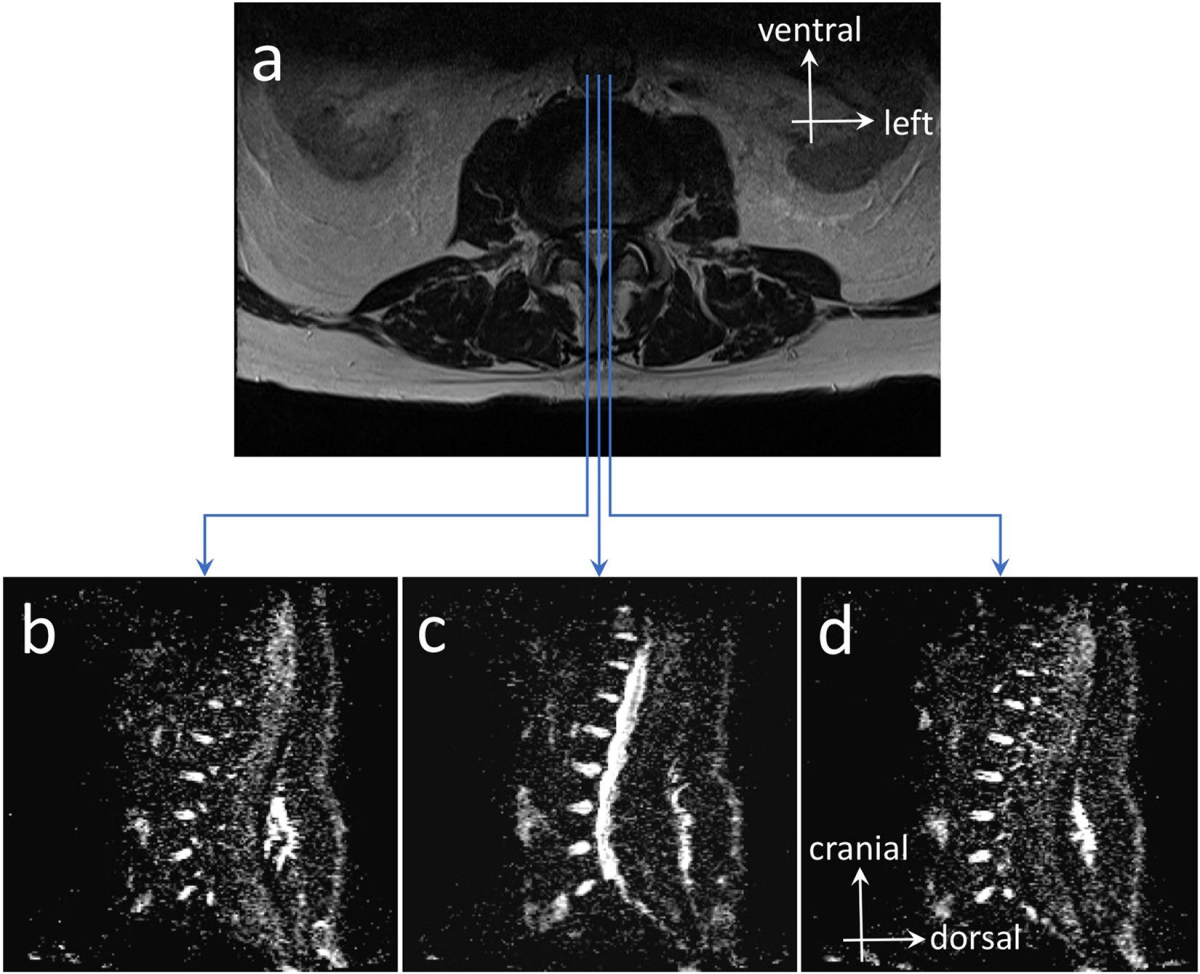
T2-weighted MRI cross section at L_4_-L_5_ IVD and $$ADC$$ mappings in sagittal medial and parasagittal planes. Position of the 3 section planes are shown on T2 image (a) and their resultant $$ADC$$ mappings in parasagittal right (b), sagittal medial (c), and parasagittal left (d) planes.
